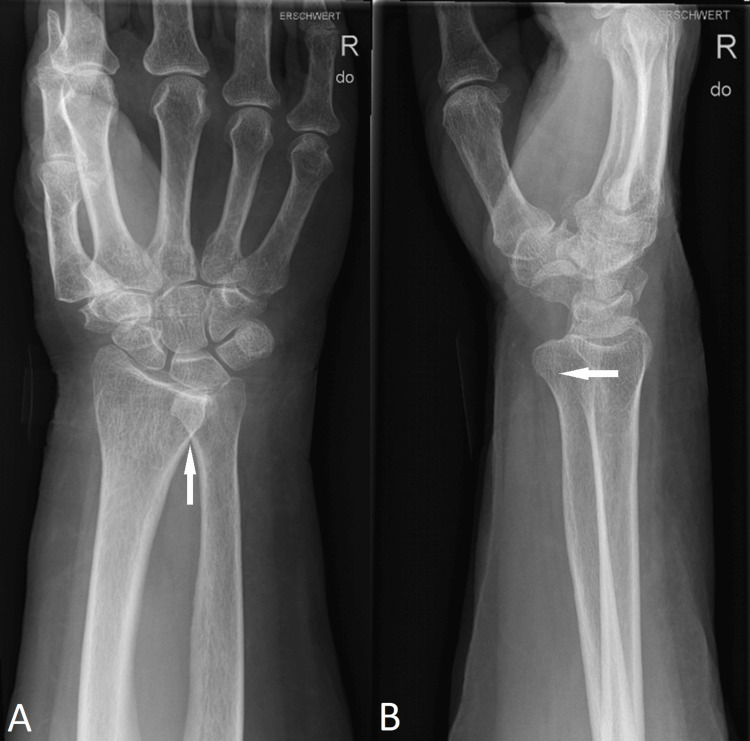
Radiographs of the right wrist showing an overlap of the distal radius and ulna (white arrow) in the posteroanterior view (A) and volar displacement of the ulnar head (white arrow) in the lateral view (B).
